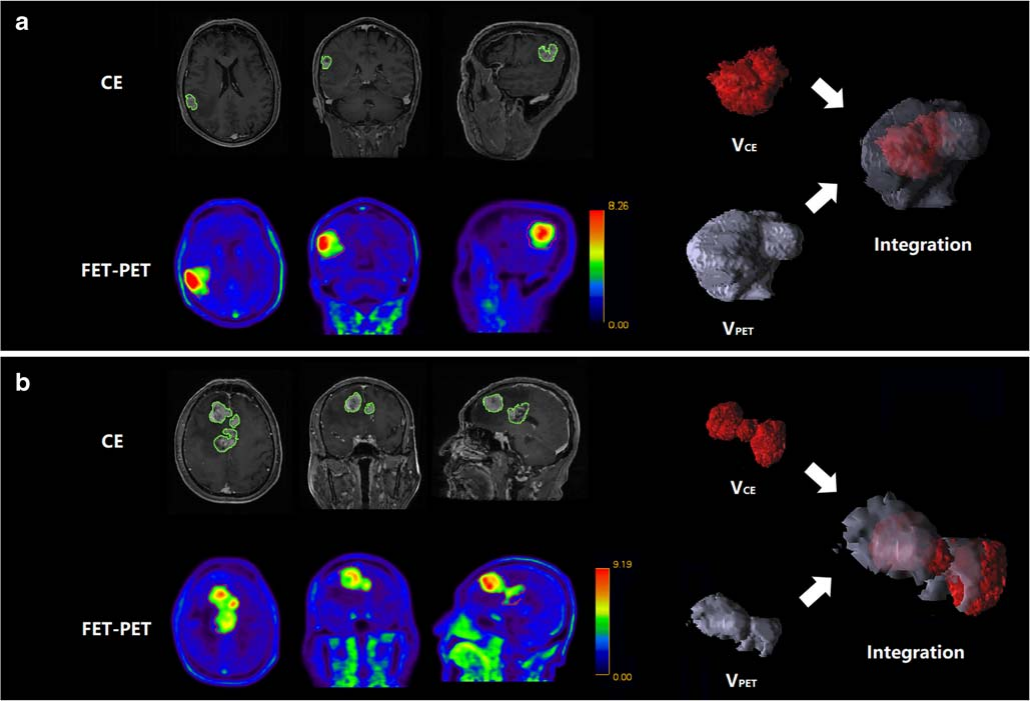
A representative comparison of *V*_CE_ and *V*_PET_ in patients 11 and 27. a Patient 11: *V*_PET_ was larger than *V*_CE_ (37.56 vs. 4.39 cm^3^). The discrepancy-CE (0.10%) and DSC (0.21) values were low, while the discrepancy-PET (88.33%) and OV values (0.99) were much higher, indicating that almost all of the *V*_CE_ was contained within *V*_PET_. b Patient 27: *V*_PET_ was larger than *V*_CE_ (56.00 vs. 38.52 cm^3^). Both the spatial similarity and overlap were relatively high (DSC 0.53, OV 0.65). However, the discrepancy-PET and discrepancy-CE (44% and 19%, respectively) were both greater than 10%, indicating that FET-PET and CE MRI complemented each other
